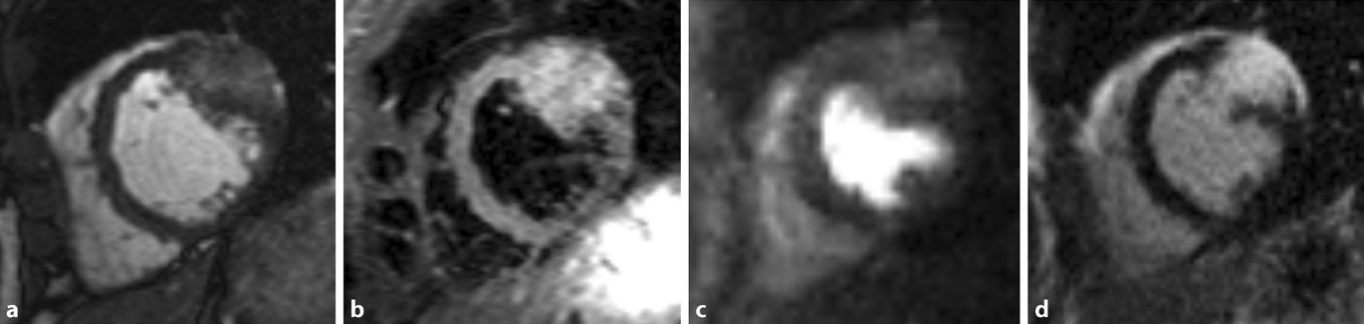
Cardiac magnetic resonance images showing a cardiac haemangioma in the short-axis view in the cine (a), T2-weighted (water) (b), first pass perfusion (c) and late gadolinium-enhancement (d) image
